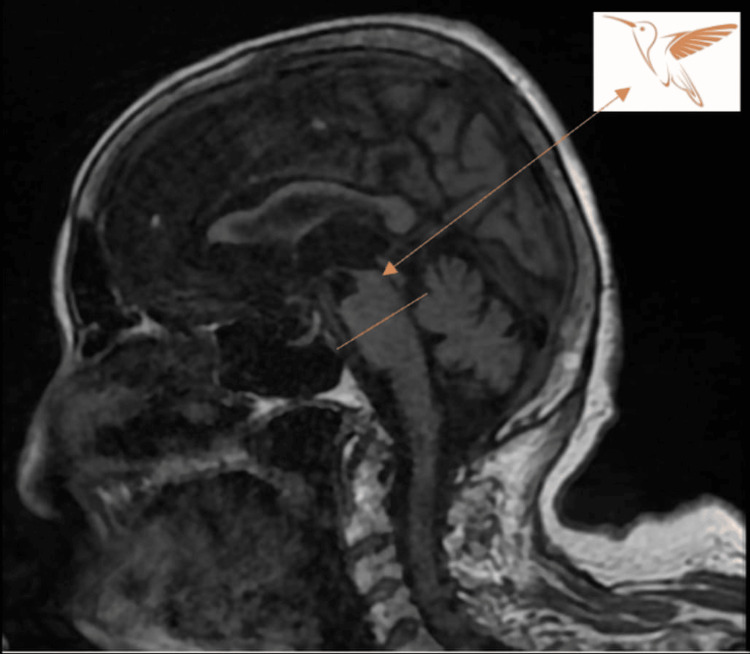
Hummingbird sign The sagittal T1 MRI image shows selective pronounced atrophy of the midbrain, with mesencephalic predominance and preservation of pons, which is concave at its cranial margin - the characteristic hummingbird sign in the PSP MRI: magnetic resonance imaging; PSP: progressive supranuclear palsy
